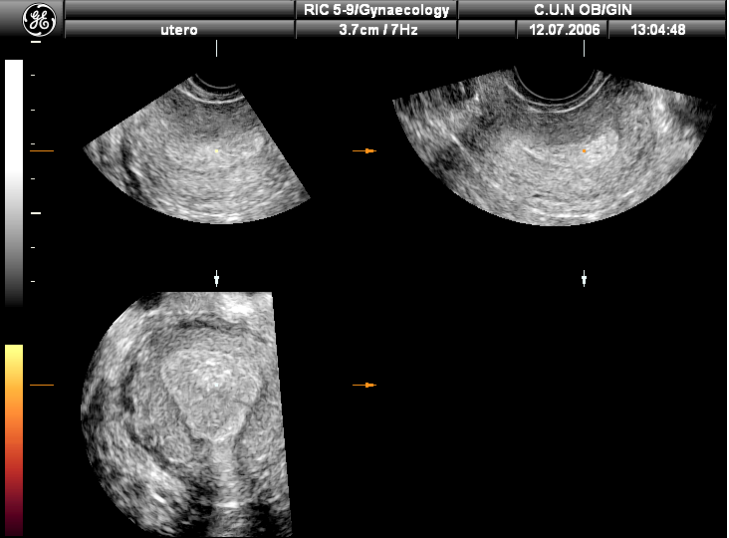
Three-dimensional ultrasound depicting multiplanar display of the uterus. All three orthogonal planes can be displayed using this technique.
